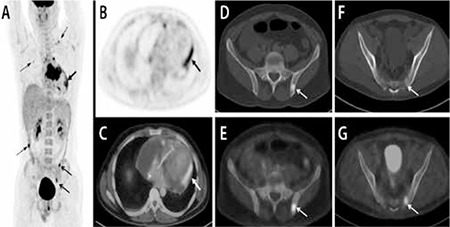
Apart from the primary tumor, PET Maximum Intensity Projection (MIP) image shows increased 18F-FDG uptake along the left lateral border of the heart (A, bold arrow) along with two foci of increased 18F-FDG uptake in left hemipelvis (arrows). Other foci of benign FDG uptake (broken arrows) on MIP image were seen in left supraclavicular region (central venous line), right hemithorax (right lung infection) and ascending colon (normal colonoscopy).Transaxial 18F-FDG PET (B) and contrast enhanced 18F-FDG PET-CT (C) show moderate pericardial effusion with increased 18F-FDG uptake in pericardium (arrow) likely infiltration (SUVmax-5.1). Minimal right pleural effusion is also noted. Transaxial CT (D) and 18F-FDG PET-CT (E) show a sclerotic lesion in left iliac bone (arrow) with increased 18F-FDG uptake (SUVmax-4.5). Transaxial CT (F) and 18F-FDG PET-CT (G) show another focus of increased 18F-FDG uptake in left ala of sacrum (SUVmax-3.7) with minimal sclerosis on CT (arrow).
